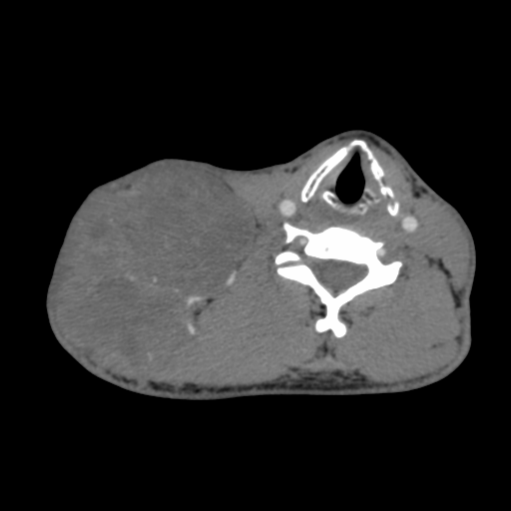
CT – laterocervical tumor
